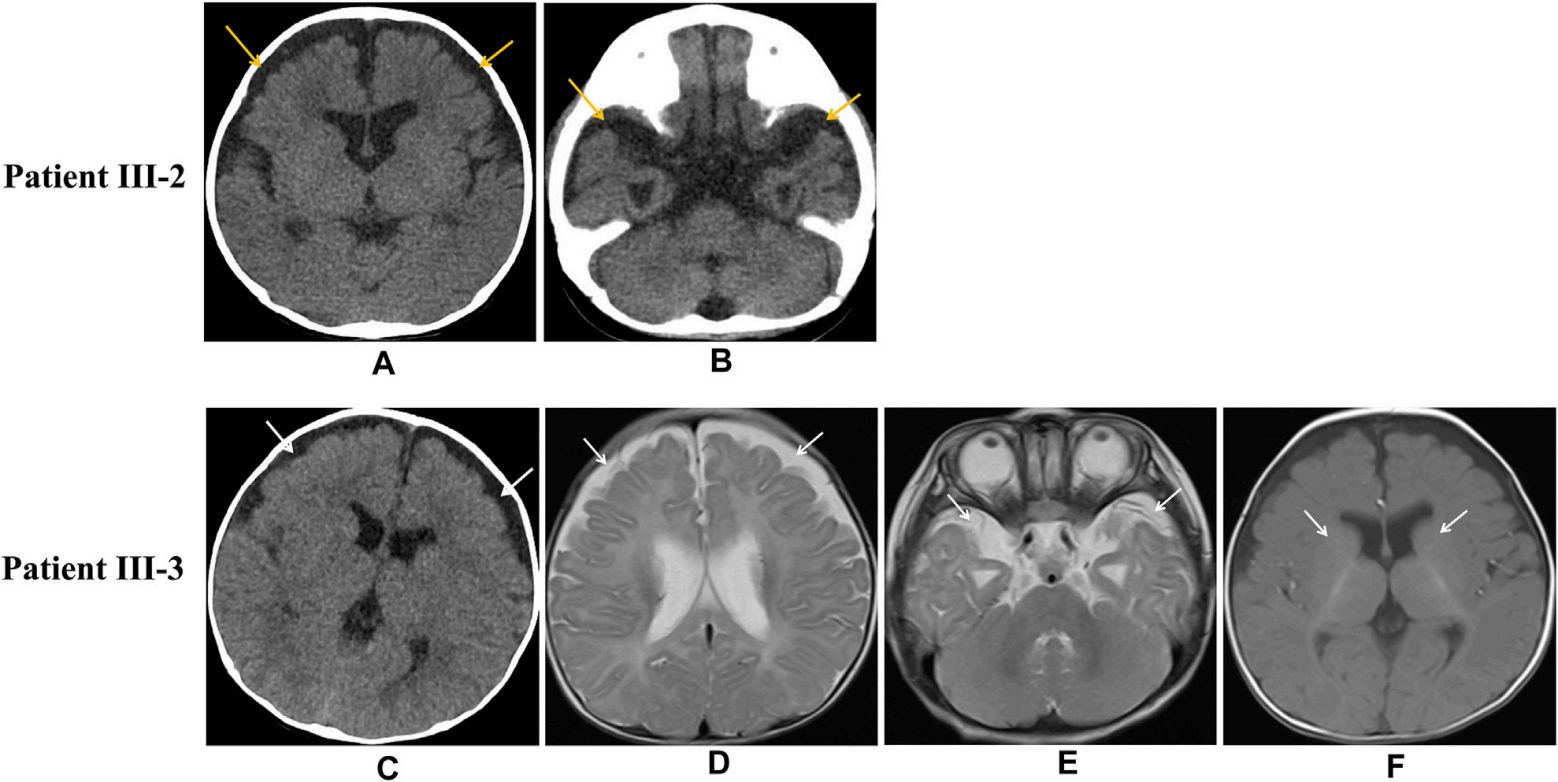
Imaging of patient III-2 and patient III-3. Unenhanced head CT indicates widening of the bifrontal frontotemporal subarachnoid space in patient III-2 (Figures 2A,B) Unenhanced head CT of patient III-3 shows bifrontal widening frontotemporal of the subarachnoid space (Figure 2C), and axial MRI shows bifrontal widening frontotemporal of the subarachnoid space (Figures 2D,E) and delayed myelination of white matter (Figure 2F).
